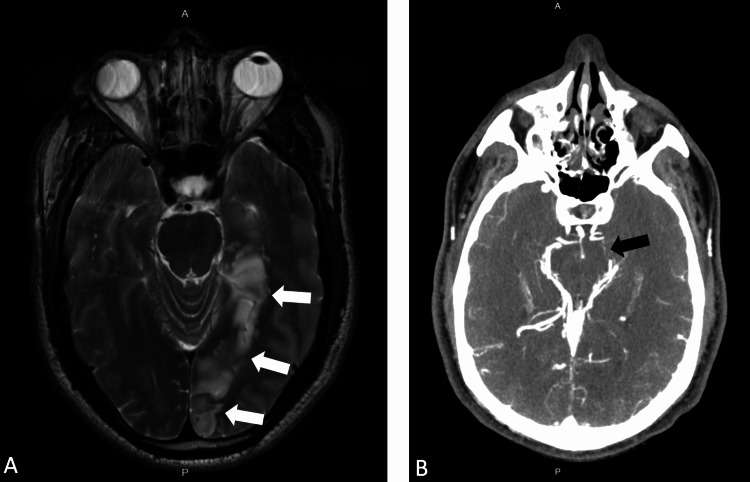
(A) Axial section of the brain MRI scan showing a large, acute left PCA territory infarction (white arrows) involving the left occipital, medial temporal, and parietal lobes, the corpus callosum, and the left thalamus. (B) CT angiogram of the head showing occlusion of the proximal P2 segment (black arrow) of the left PCA A: anterior, P: posterior, MRI: magnetic resonance imaging, CT: computed tomography, PCA: posterior cerebral artery
